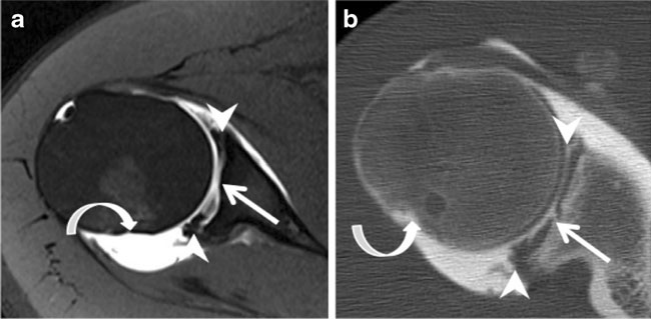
Global labral (SLAP IX) tear in a 24-year-old male athlete with a 10-month history of right shoulder pain. Axial (a) fat suppressed T1-weighted MR arthrography and (b) corresponding axial CT arthrography images. Cartilage defect (*straight arrow*) is more conspicuous on CT arthrography than on the MR arthrography image. Circumferential labral abnormalities (*arrowheads*) extending across the labro-bicipital complex (not shown) in keeping with SLAP tear type IX. A subtle osseous impaction of the posterosuperior aspect of the humeral head (Hill-Sachs defect) (*curved arrow*) is detected suggesting prior anterior dislocations
